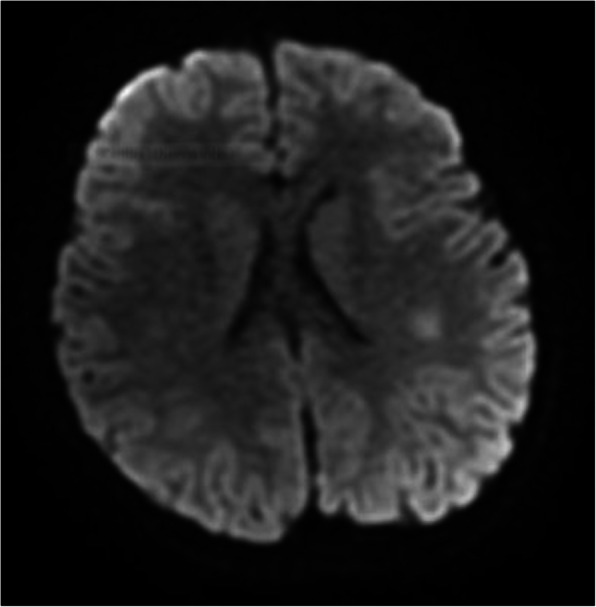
MRI DWI: lesion in the left subcortical white matter
